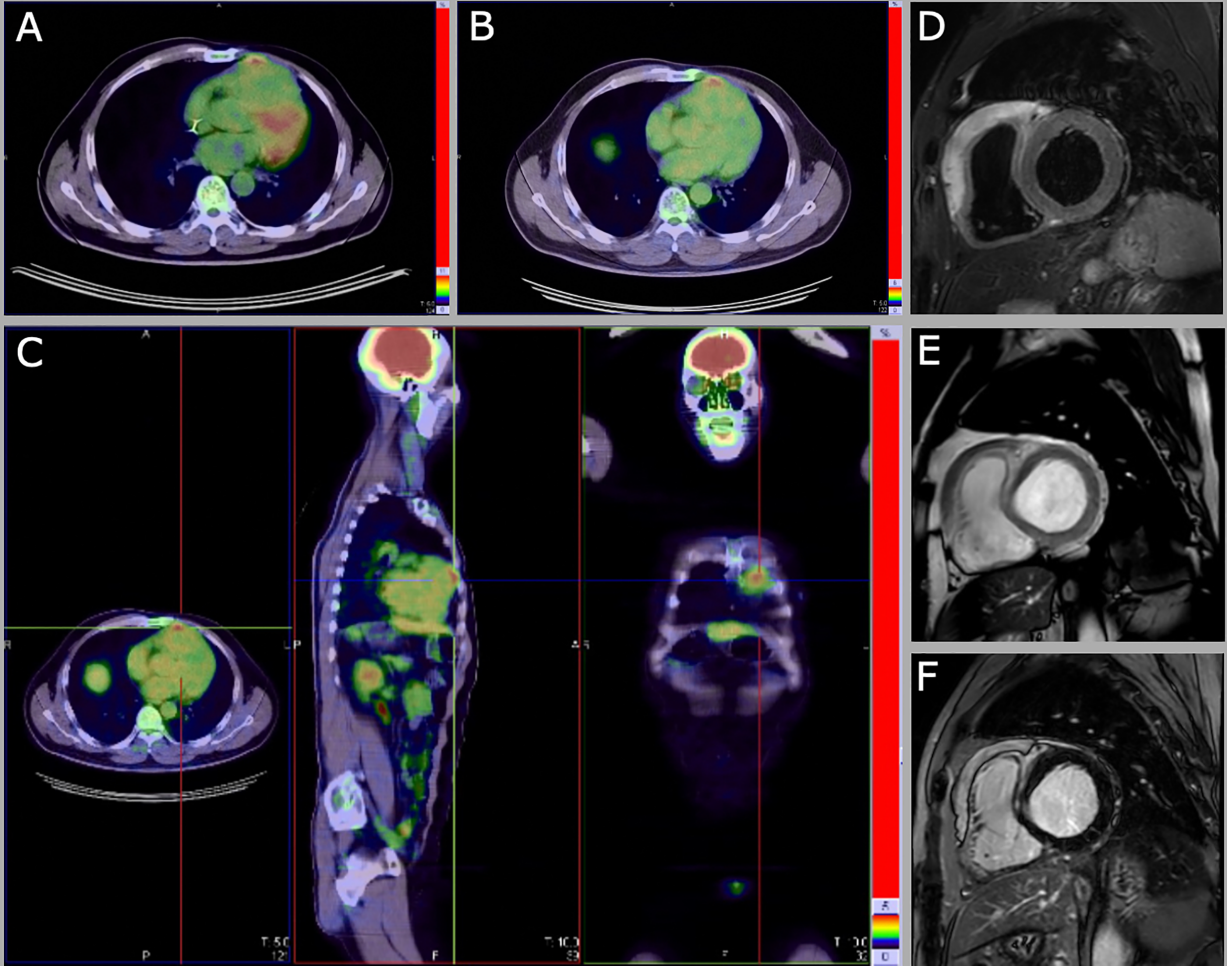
CT-PET abnormal 18-FDG uptake on right ventricle thickening 3 months (SUV 4.9, DS 4) (A) and 18 months (SUV 5 – DS 4) (B, C) after ASCT. Cardiac MRI images of June 2019 (from D to F) show the persistence of the right ventricle anterior wall thickening (14x56 mm). (D) Short tau inversion recovery (STIR) T2 black blood image in short axis on the cardiac base. (E) Steady-state free precession (SSFP) balance image with T2/T1 weighting in short axis on the cardiac base. (F) IR TSET1 image for evaluation of myocardial late enhancement in short axis on the cardiac base.
